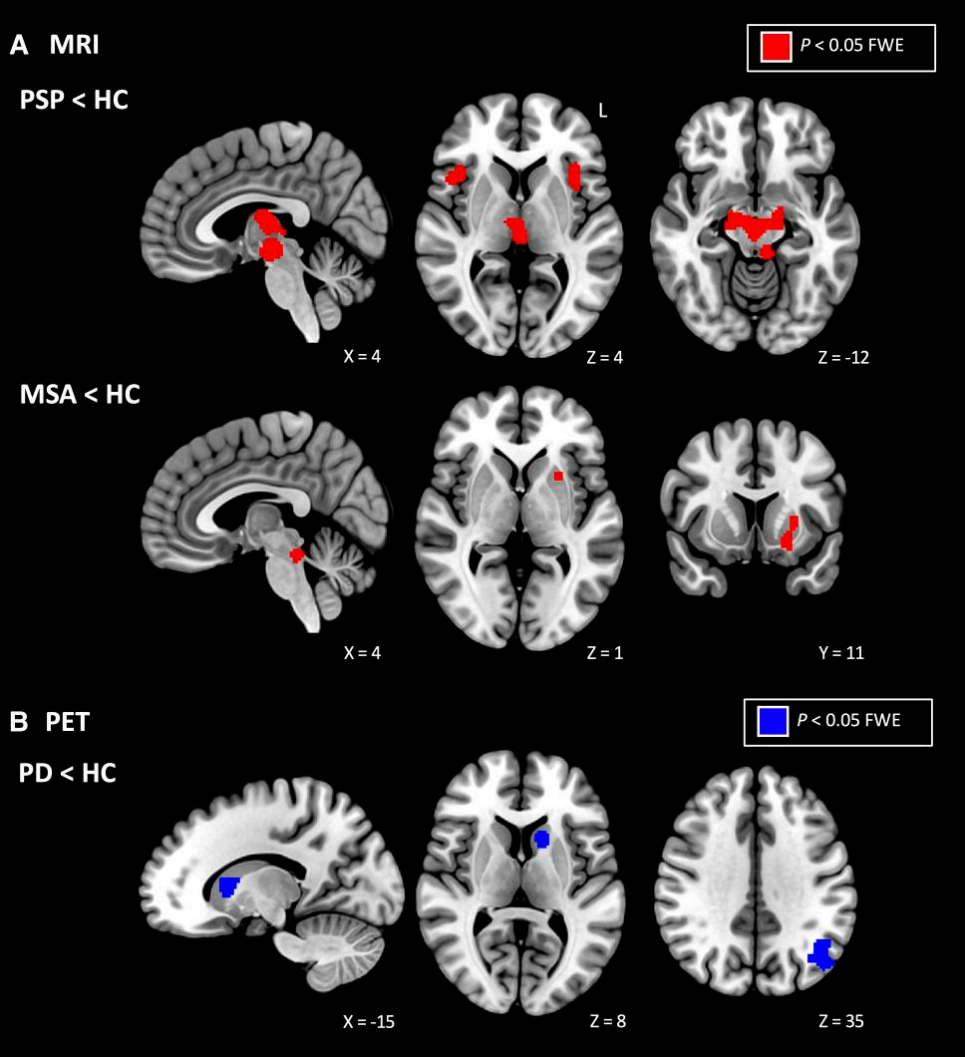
Meta-analysis results in individual parkinsonian disorders. (A) Meta-analyses of MRI imaging, patients < HC. Alteration of the red nucleus was characteristic of PSP patients, whilst the brainstem and putamen were the most commonly implicated regions in patients with MSA. (B) Meta-analysis of PET imaging in Parkinson’s disease; patients < HC. The middle temporal gyrus and left caudate were consistently implicated by PET imaging in Parkinson’s disease patients. All significant clusters are reported in Table 3. FWE = family-wise error; L = left; MSA = multiple system atrophy; PD = Parkinson’s disease; PSP = progressive supranuclear palsy.
